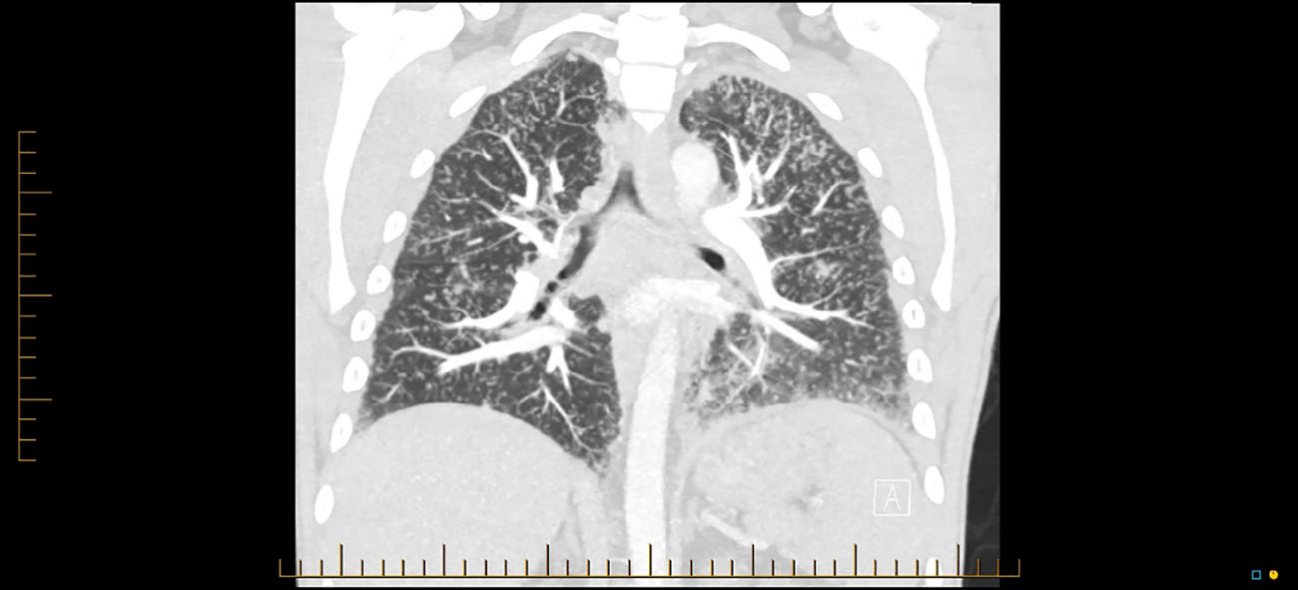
Computed tomography pulmonary angiogram with disseminated miliary micro‐nodularity
